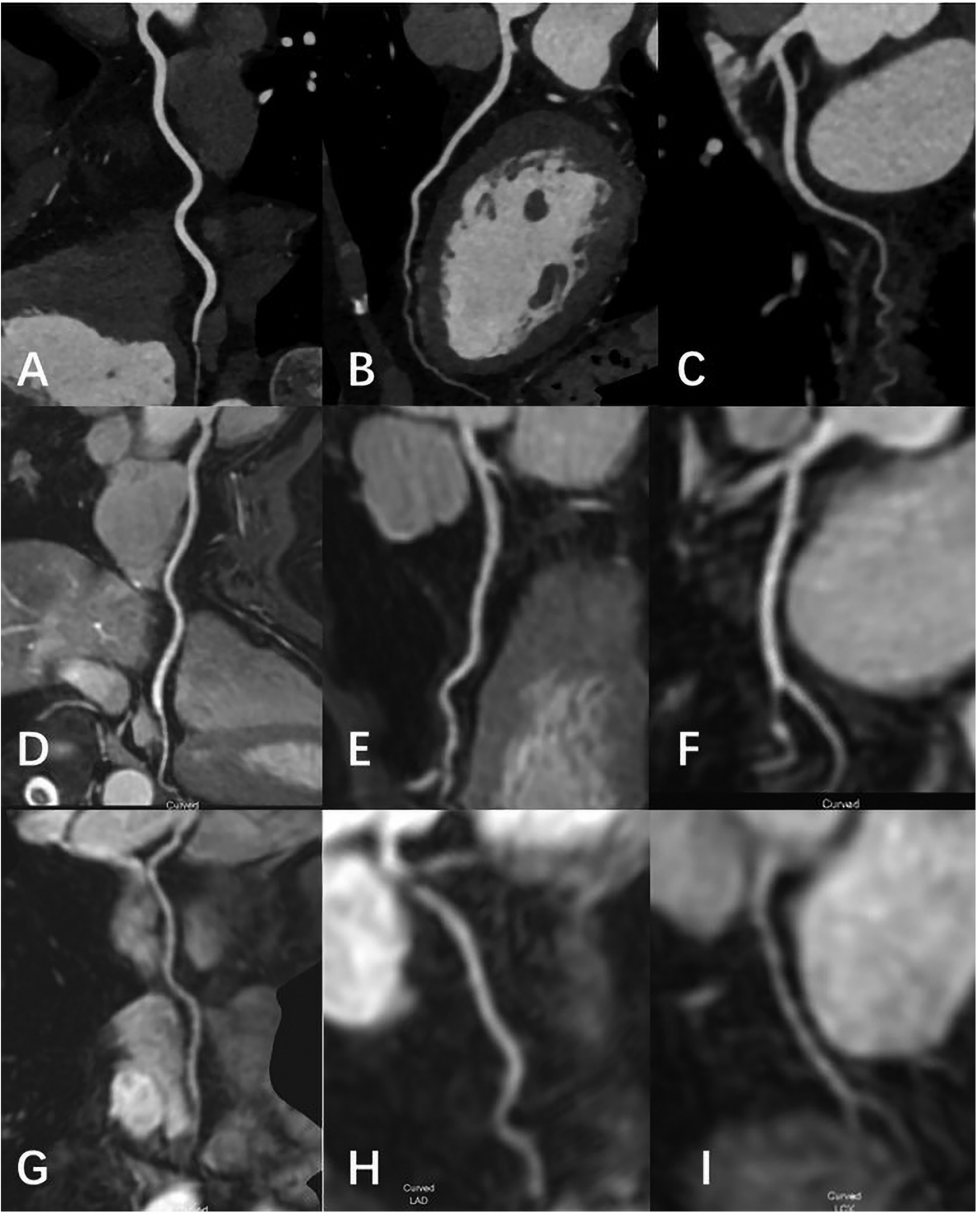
Female, 69 Y, with chest pain. (A–C) The RCA, LAD, LCX shown in coronary CTA, coronary artery stenosis was not observed; (D-F) the RCA, LAD, LCX shown in 3D-BTFE sequence, the lumen edges were sharp, the coronary arteries were clearly delineated, and the subjective images scored 5; (G–I) the RCA, LAD, LCX shown in MSG-EPI sequence, and the subjective images scored 4.
